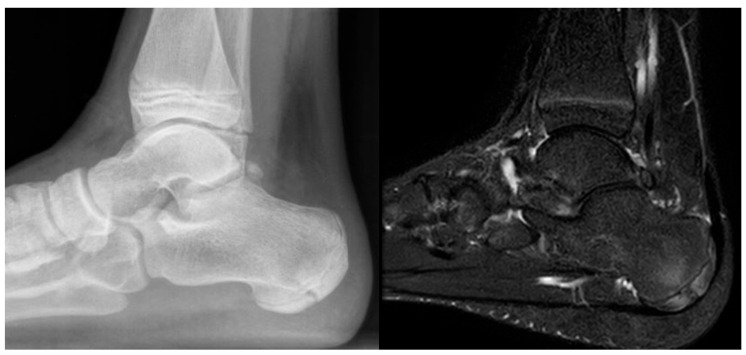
Eleven-year-old male patient. Radiograph (left) demonstrating typical fragmentation and bipartite appearance of the calcaneal apophysis. MRI (right) showing detailed fragmentation with associated bone marrow edema.
